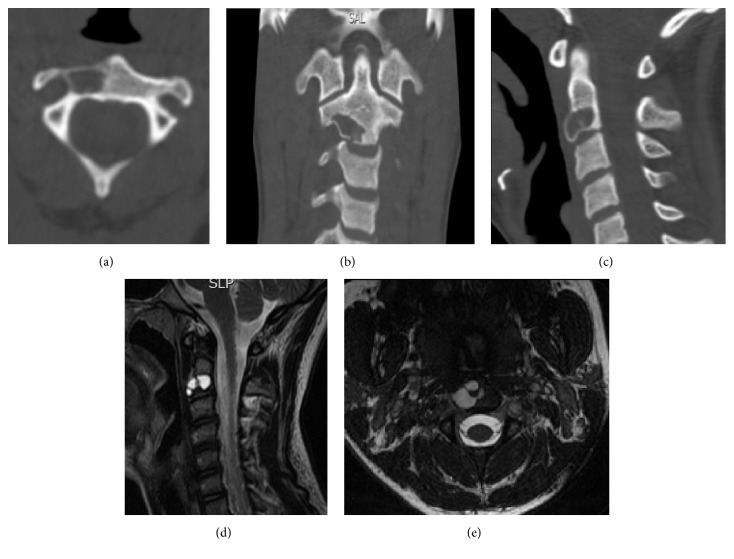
(a), (b), and (c) CT Lesion with cystic appearance, defined borders compromising two-thirds of body C2, in close proximity to the right vertebral artery. (d) MRI of the lesion. (e) Axial sequence of the preoperative MRI.
